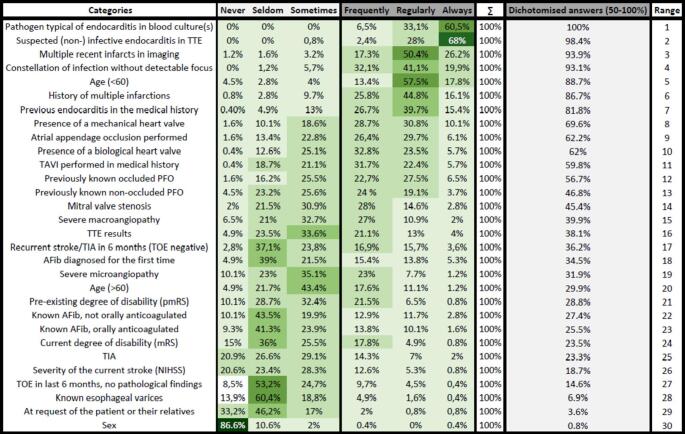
Impact of different findings on the decision to perform transoesophageal echocardiography (TOE) in stroke units; The dichotomized answers include all responses marked as ‘frequently,’ ‘regularly,’ or ‘always’ (representing 50–100% in the questionnaire). These dichotomized values are moreover ranked by their importance as provided by the responding stroke unit leads (i.e., 100% of leads reported that a pathogen typical of endocarditis in blood culture(s) should be an indication for TOE)
